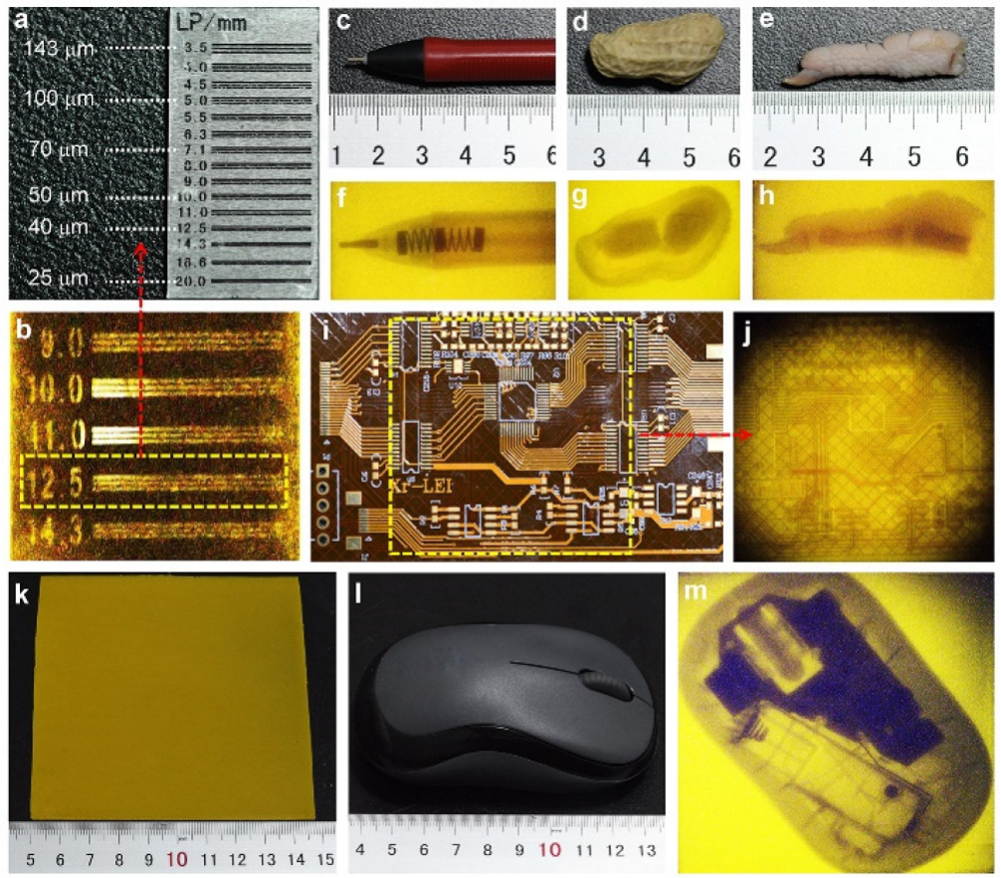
(a) X-ray high-resolution linear mask and corresponding
